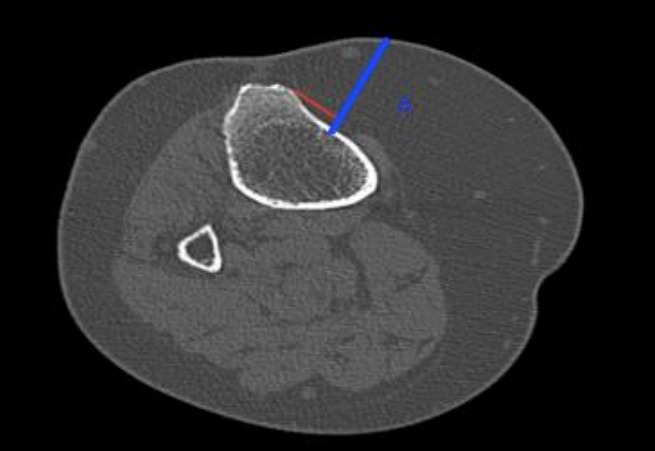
Radiographic representation of the pre-tibial soft tissue depth. The blue line represents tissue depth (i.e., perpendicular distance from the appropriate intraosseous insertion site to the skin surface, at a right-angle from the plane of the bone surface). The red line represents a line parallel to the surface of the tibia.
